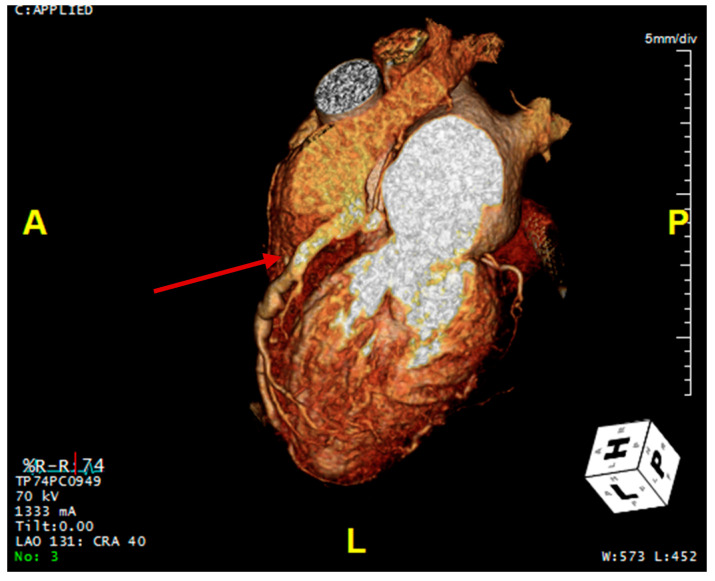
Coronary CT angiogram demonstrating the dilated right coronary artery (the right coronary artery is marked by arrow).
